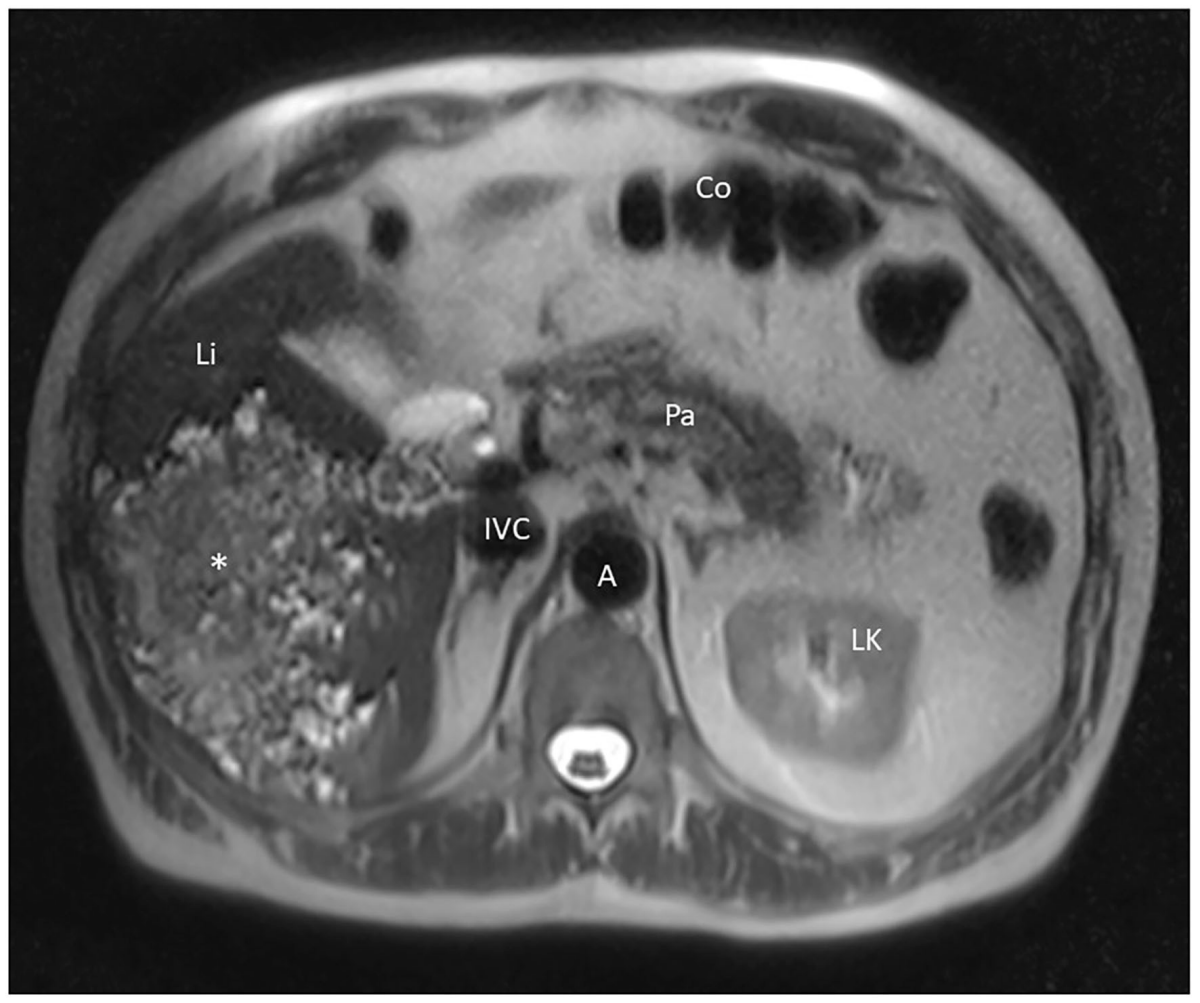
MRI imaging showing the AE lesion of our patient in the axial plane. Li, liver; Co, colon; Pa, pancreas; LK, left kidney; A, aorta; IVC, inferior vena cava, *AE tumor.
